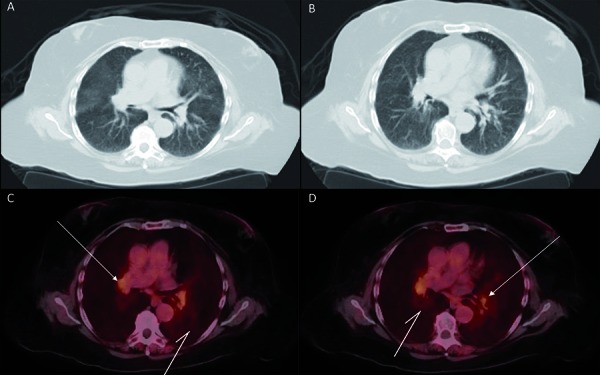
A, B: High-resolution computed tomography (perihilar slice) showing normal lung parenchyma and without any lymphadenopathy; C, D: Positron emission tomography scan with flourodeoxyglucose (FDG) showing right upper lung lobe lymphadenopathy (white two-pronged arrows) and increased FDG uptake of lung parenchyma (white one-pronged arrows).
